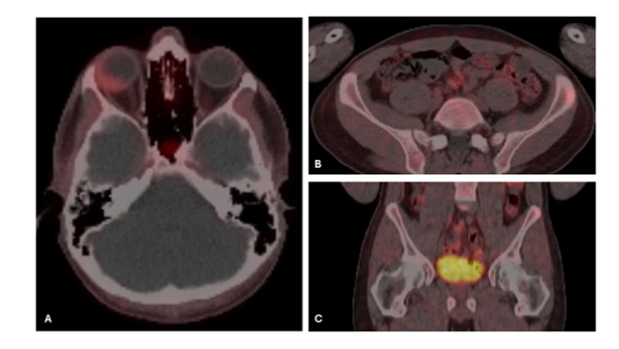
DOTATATE PET Imaging on d 64 demonstrating sites of metastatic involvement(A) Axial DOTATATE PET of the orbits showing mild radiotracer avidity within the right choroidal lesion (SUV 2.6; Krenning score 1), corresponding to the enhancing plaque previously identified on MRI; (B) Axial PET of the pelvis showing a mildly avid lytic lesion in the left iliac crest (approximately 1 cm), consistent with metastatic involvement; (C) Coronal PET confirming the left iliac crest lesion with focal uptake.
